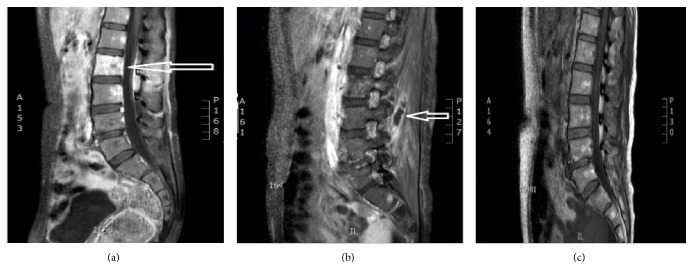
MRI lumbar spine. (a) MRI of the lumbar spine with contrast showing L2 vertebral osteomyelitis. (b) MRI of the lumbar spine showing paraspinal abscess. (c) Follow-up MRI of the lumbar spine showing resolution of the osteomyelitis and the paraspinal abscesses.
